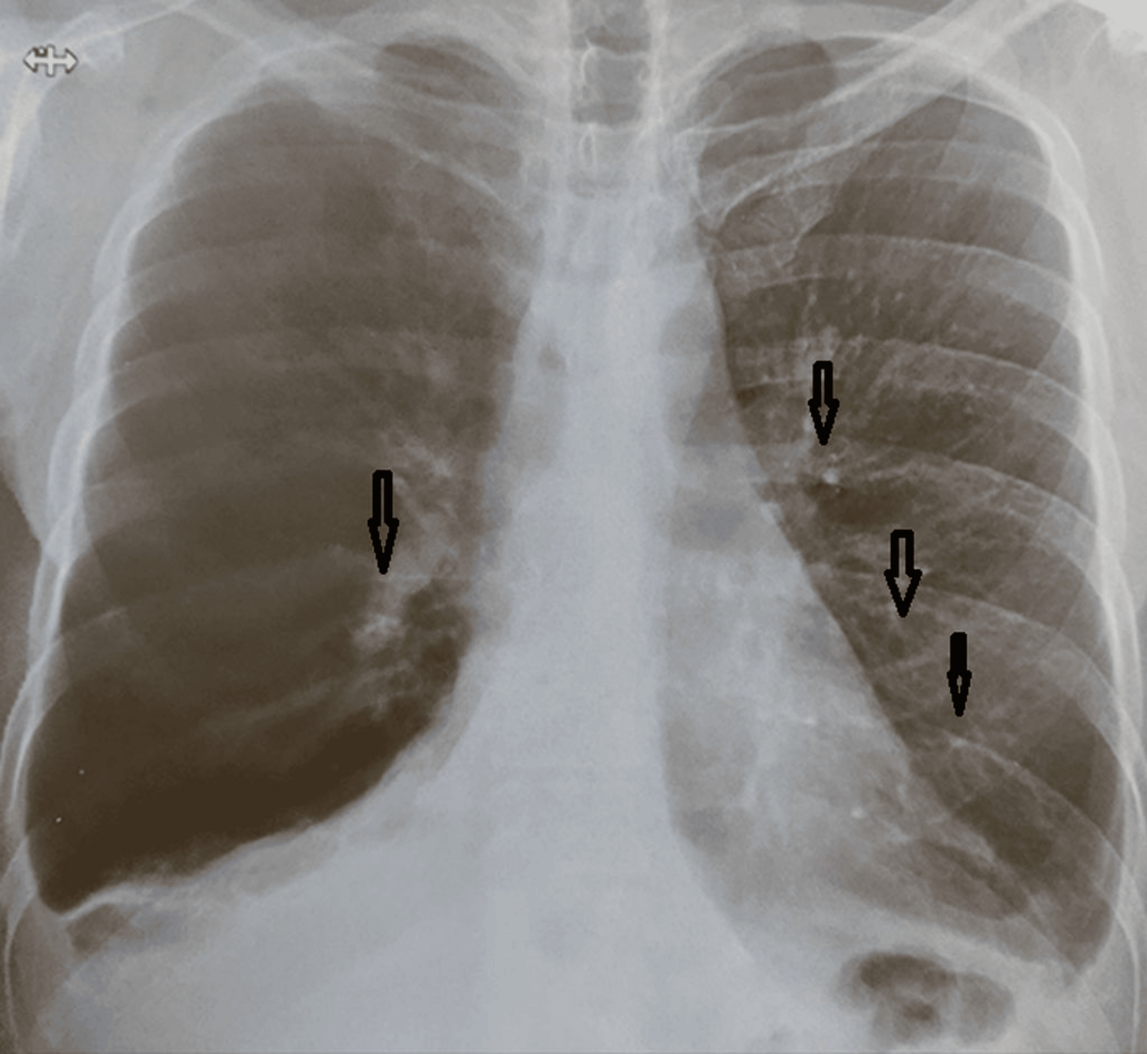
Chest X-ray The chest-pleuro-mediastinal-cardiopulmonary radiograph upon emergency presentation.
